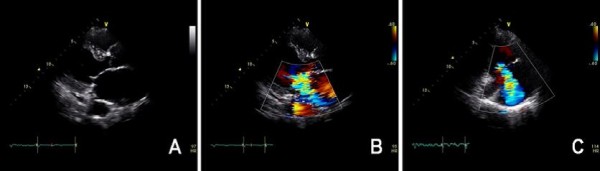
Preoperative transthoracic echocardiographic images. (A) The parasternal long-axis view shows the prolapsed anterior leaflet of the mitral valve, while (B) color flow mapping reveals severe regurgitation of the mitral valve. (C) The modified two-chamber view with color flow mapping reveals moderate to severe regurgitation of the tricuspid valve.
